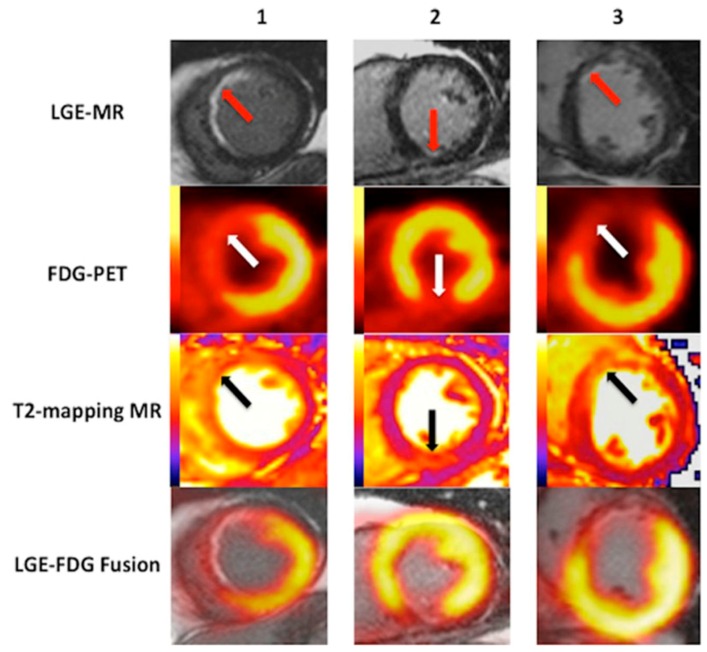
Hybrid PET/CMR imaging of three subendocardial infarctions (*red arrows*) with significant myocardial salvage. In all of the studies, the areas of reduced ^18^F-FDG uptake (*black arrows*) and of increased T2 mapping (*white arrows*) extended beyond the areas of LGE. In Study 2, the area of reduced ^18^F-FDG uptake is substantially larger than the area of LGE and more closely matched the T2 map. The follow-up scan confirms the presence of myocardial salvage, in which in area of reduced ^18^F-FDG uptake decreases in size and matches the areas of infarction. Reproduced from Bulluck et al., 2016, by permission of *Circ. Cardiovasc. Imaging* [123].
